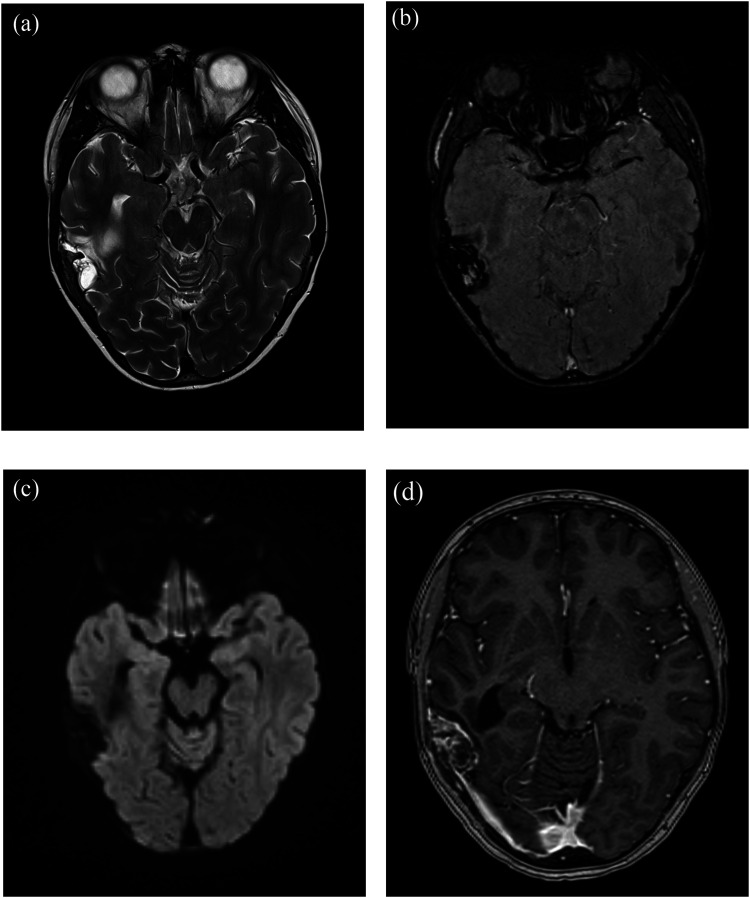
Follow-up MRI, 4 months after the initiation of neoadjuvant chemotherapy and before surgery, shows full regression of extracranial soft-tissue mass and partial regression of the intracranial soft-tissue mass. The intracranial component showed areas reminiscent of ABC-likes changes (blood-filled spaces with hemosiderin contain) on axial T2W imaging (a) and on axial susceptibility weighted imaging (SWI) (b). No areas of high signal intensity on axial DWI (b = 1000 s/mm^2^) (c), and septal and rim contrast enhancement near temporal bone on axial postgadolinium T1W imaging (d), indicating some viable residual tumor tissue.
